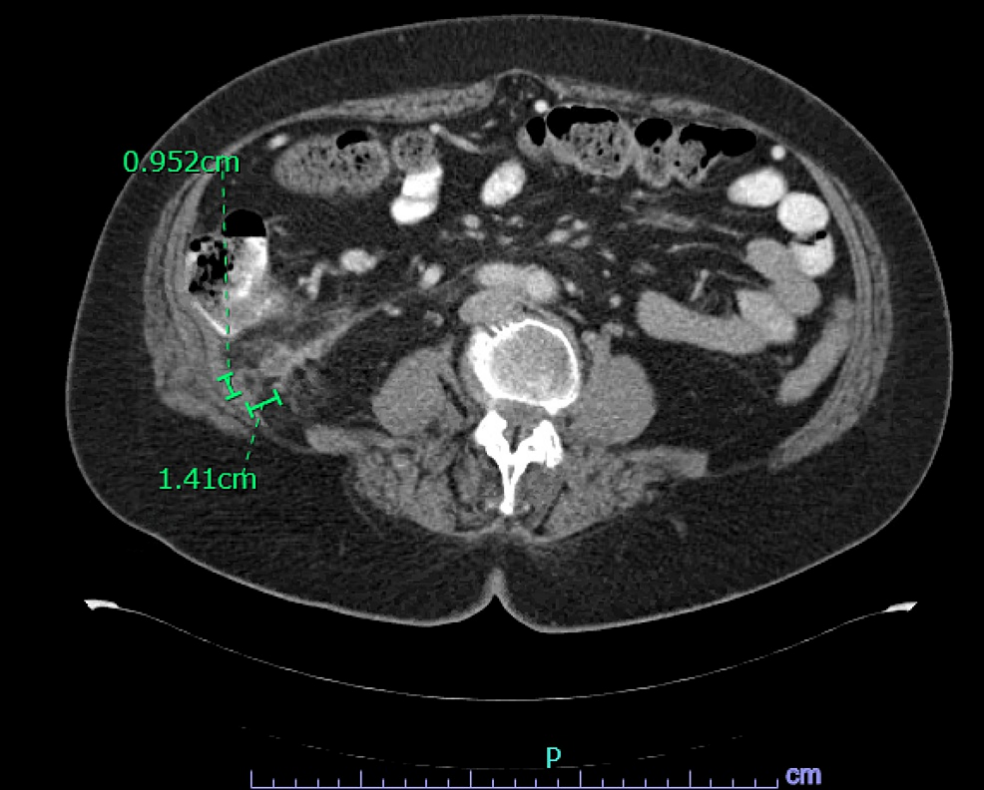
CT abdomen and pelvis with contrast showing posterior to the hepatic flexure along the inferior aspect of the right hepatic lobe. There is a focal area of fat stranding with an associated fluid collection measuring 1.4 x 1.0 x 1.2 cm. There is no significant colonic wall thickening.
